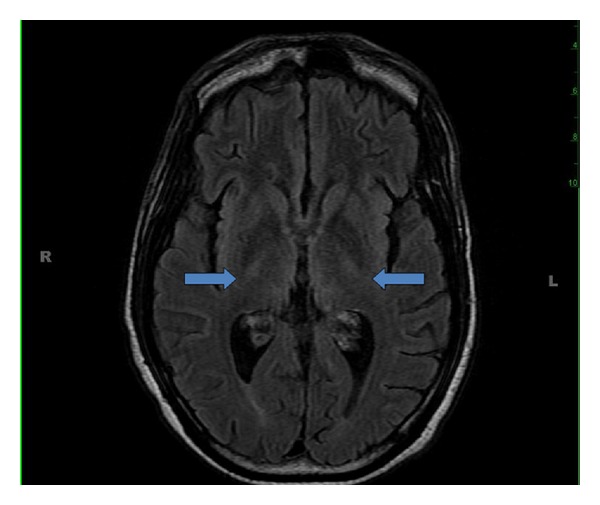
MRI demonstrating slight increase in T2 signal in the medial bilateral thalamus (arrows).
